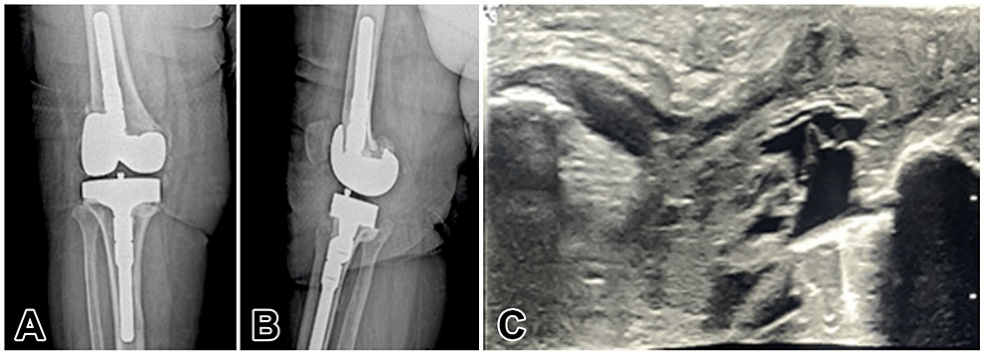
A: Anteroposterior knee radiograph of the side with preoperative patellar tendon rupture. B: Lateral knee radiograph of the side with preoperative patellar tendon rupture. C: Patellar tendon rupture USG image USG, ultrasonography.
